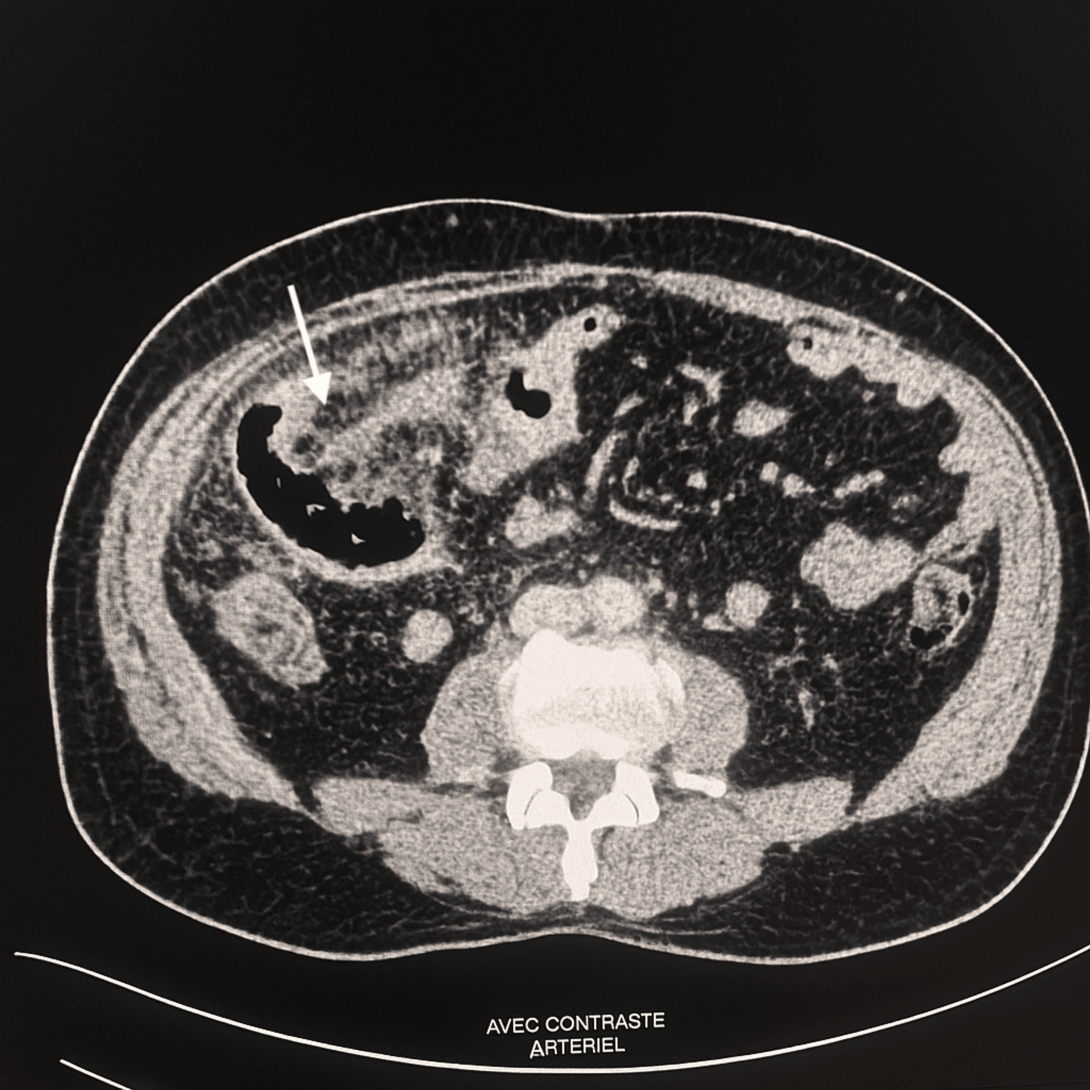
An axial slice from an abdominal CT scan with arterial contrast injection showing terminal ileal diverticulitis (arrow)
